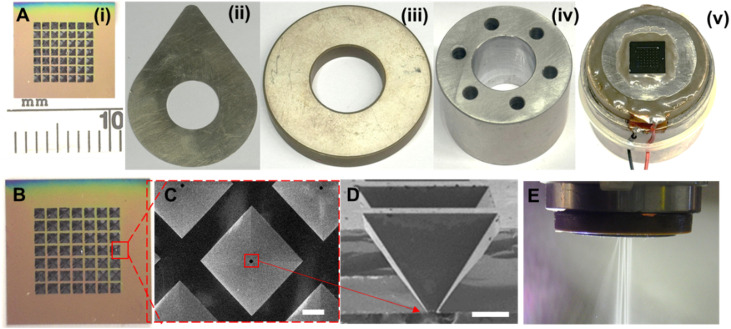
Assembly of the microparticle production head components; (A) images of microparticle producer head components: (i) microfabricated spray nozzle array, (ii) metal disc, (iii) ultrasonic (piezoelectric) transducer, (iv) solution reservoir, (v) assembled microparticle production head. (B) A magnified image of a microfabricated spray nozzle array. (C and D) SEM image of a single nozzle (scale bars show 200 μm). (E) Image of an operating microparticle production system.
